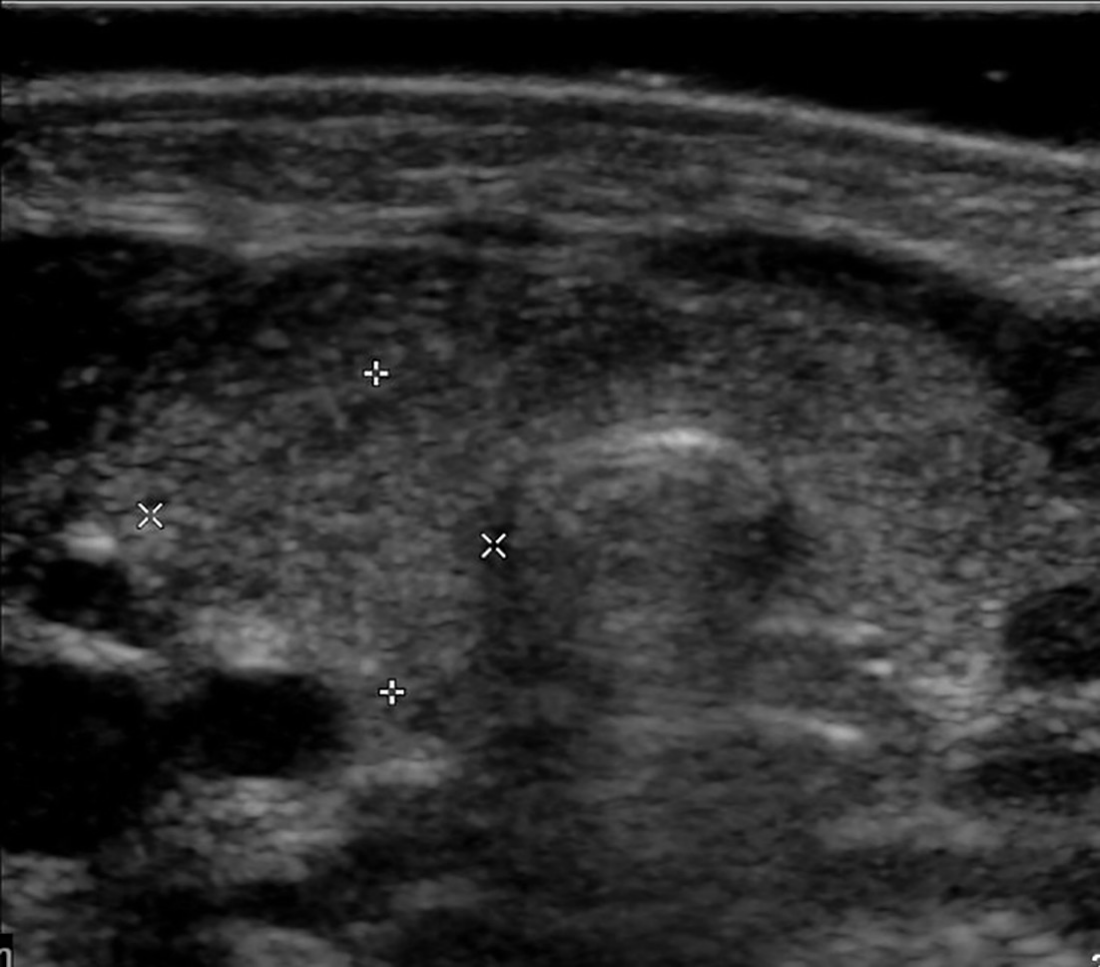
Thyroid ultrasound in an infant with Down syndrome and *PAX8* mutation (patient P11 in Table 1 and Hermanns et al). Thyroid appears bulky but combined lobe volume is actually small at 0.78 ml (reference range 0.8–2.4 ml).
